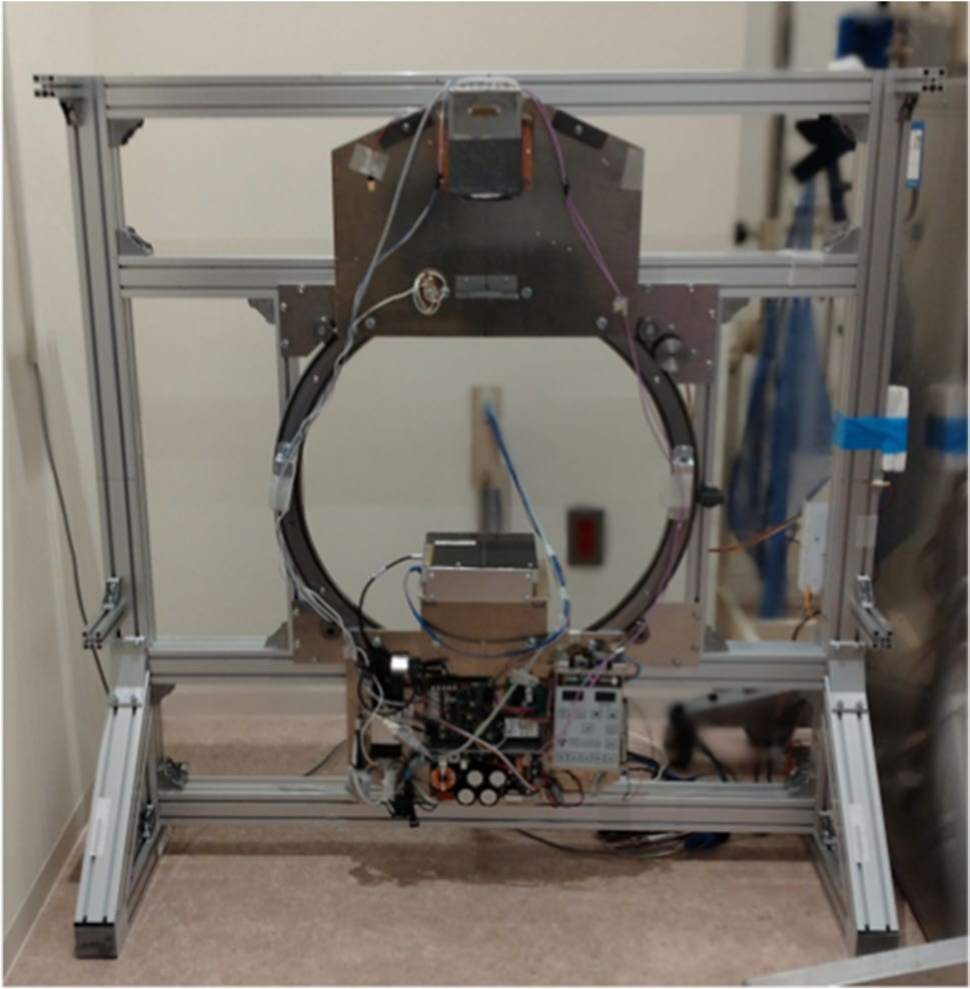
An ultra-high-resolution cone-beam CT system (UHRCBCT) for hand and foot we developed
